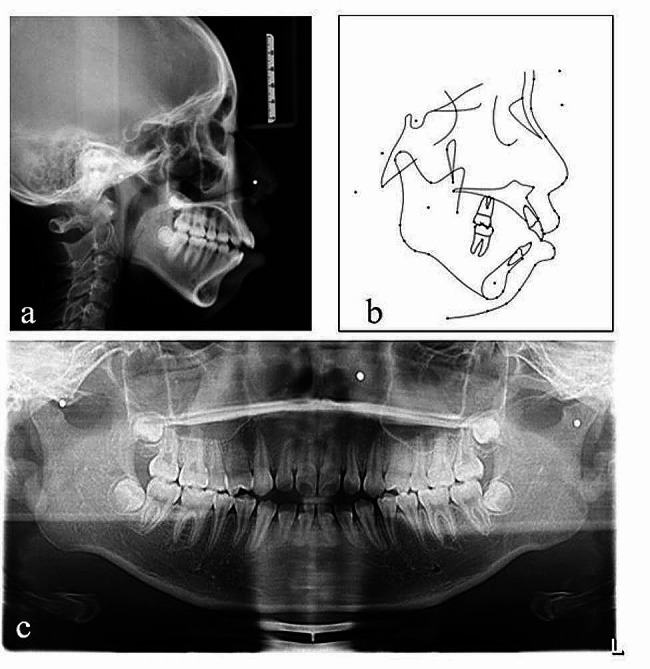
Pretreatment radiographs: a lateral cephalogram, b Pretreatment lateral cephalogram tracing, c panoramic radiograph
